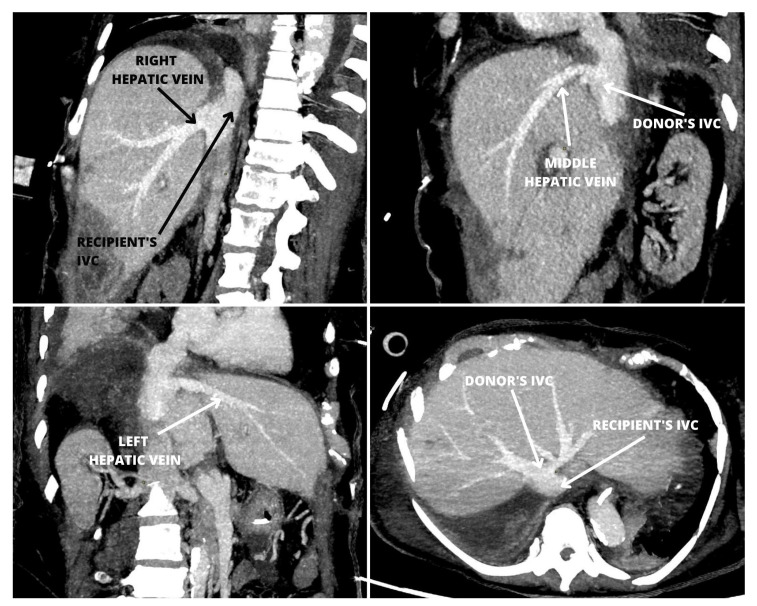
Computed tomography of a patient after liver transplantation with a sutured side-to-side inferior venae cavae anastomosis shows an anatomy similar to a stapled anastomosis.
